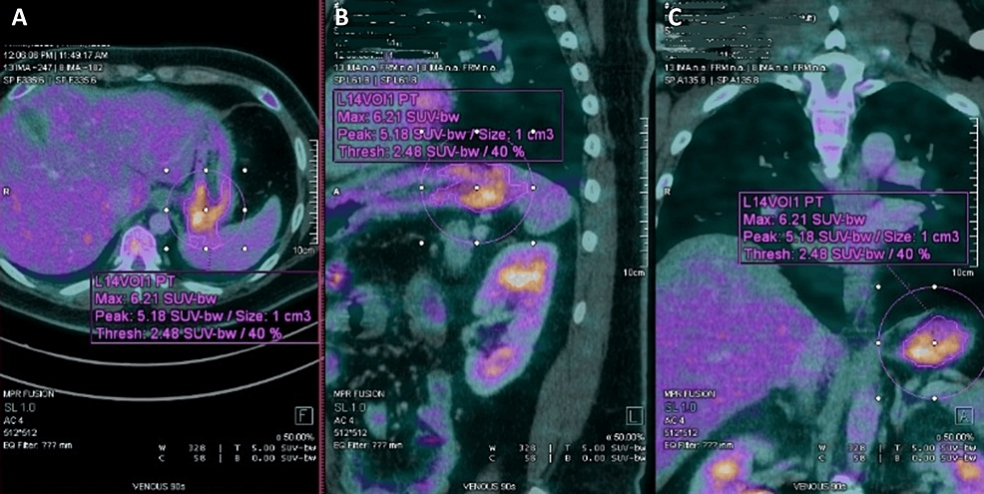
Whole-body positron emission tomography-computed tomography (PET-CT) excluding the brain. A presacral soft tissue component and a moderately hypermetabolic soft tissue mass (circle) involving the S1–S3 vertebral bodies can be observed (SUVmax: 6.16) at the coronal (A), lateral (B) and anterior-posterior views (C), which is strongly suggestive of a malignant process.
